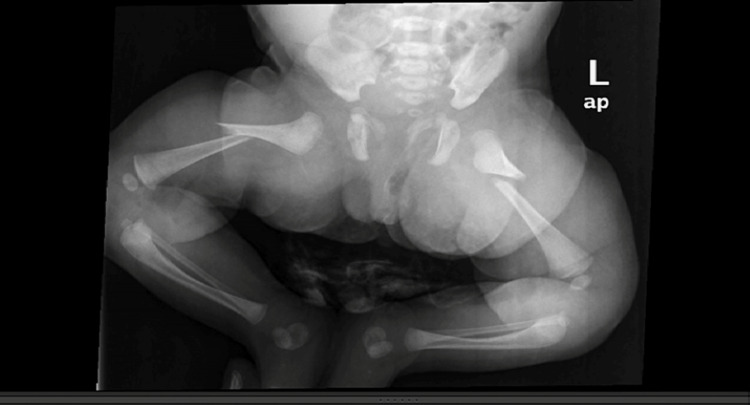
Radiography from the emergency room showing a bilateral shaft of the femur fracture.
